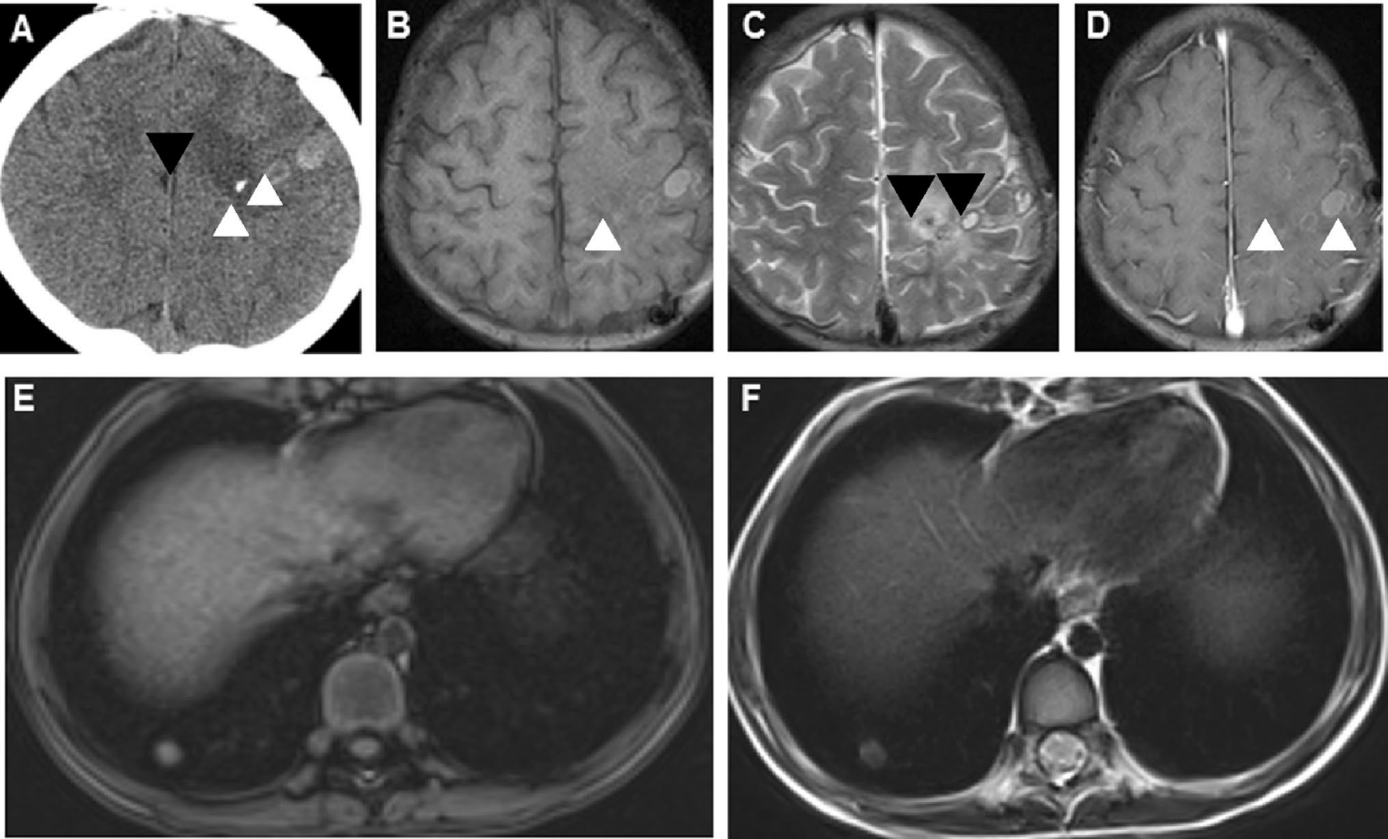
A 4-year-old boy presented with paroxysmal headache and vomiting. (A–D) Characteristic ring-like lesions with surrounding edema (white arrowhead) and calcifications (black arrowhead) located in the left frontoparietal lobe showed by cranial CT (A), T1WI (B), T2WI (C) and enhanced MRI (D); (E–F) Chest MRI indicated the nodules in the lower lobe of the right lung.
